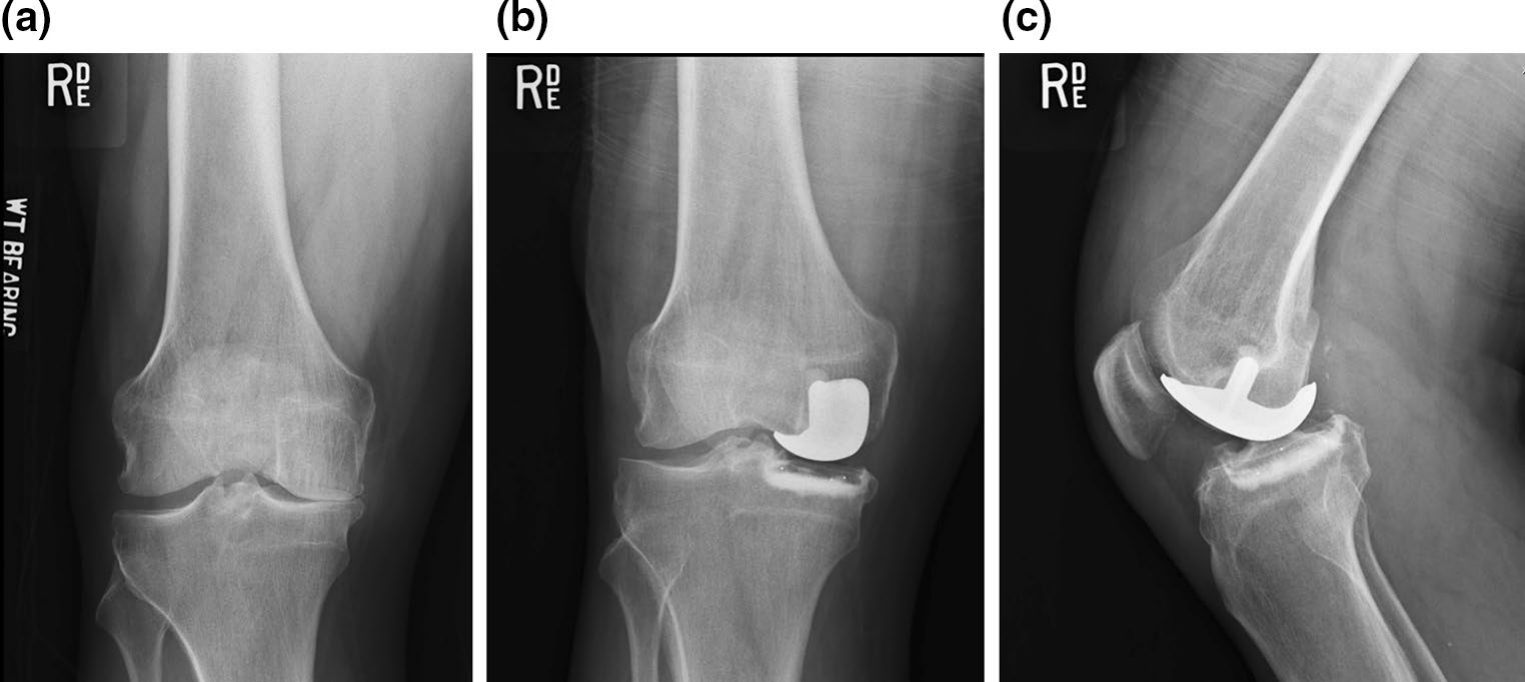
a 58-year old male patient with presenting with medial pain. Preoperative anteroposterior radiograph. b Postoperative anteroposterior and lateral radiographs. Postoperative radiographs show correct implant position. c Lateral radiograph
